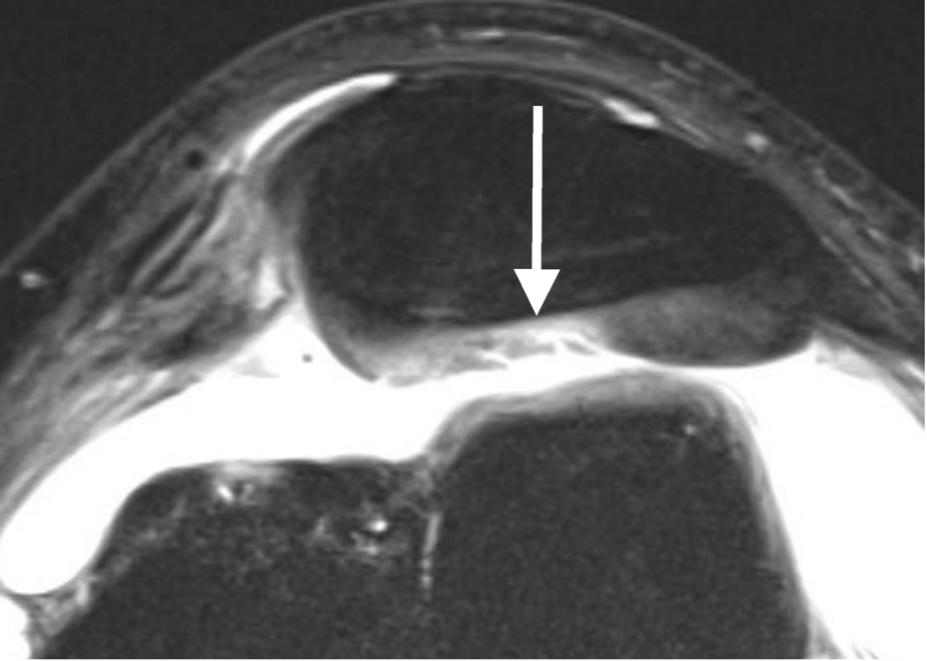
Axial PD-weighted TSE MRI of a 29-year-old male with a first LPD. A defect of > 50% of the retropatellar cartilage is depicted (white arrow). This finding is defined as a grade 3 disorder.
